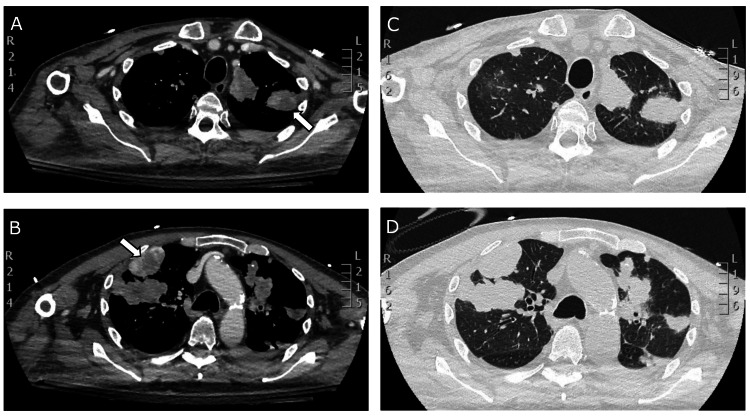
Axial chest CT images demonstrating bilateral subpleural metastatic lesions with central necrosis (arrows) on day 10 following the resolution of pneumothoraces and chest tube removal (A, B: soft tissue window; C, D: lung window). CT: computed tomography
